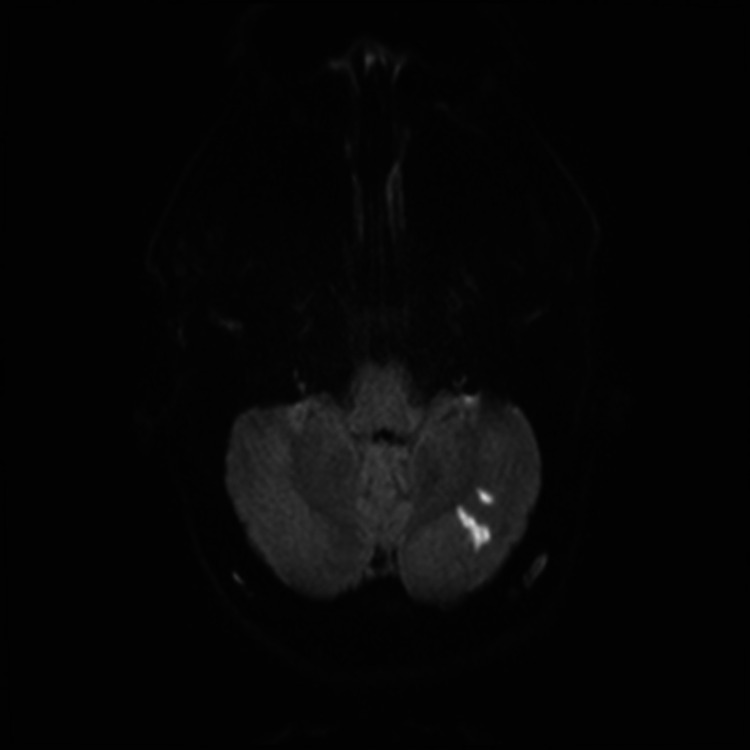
MRI of the brain with diffusion-weighted images (DWI) shows small infarctions at the left cerebellum.
